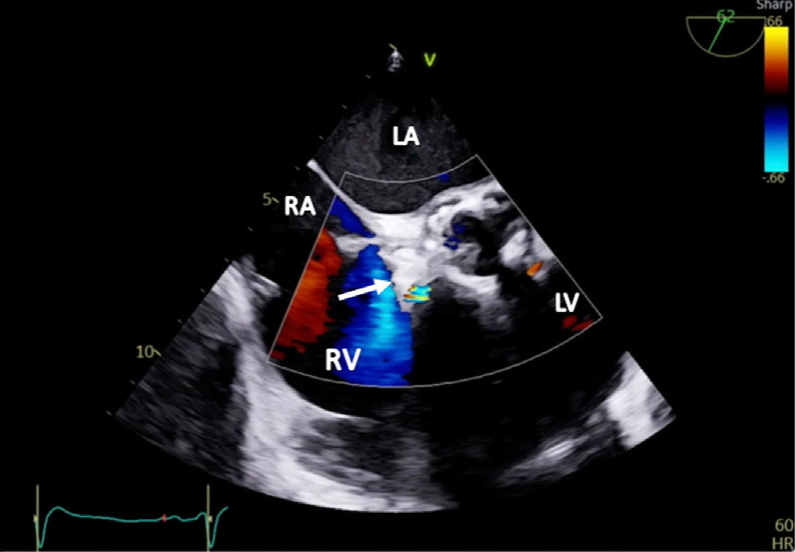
Transesophageal Echocardiographic Short-Axis View at the Aortic Valve Level With Color Flow Doppler Showing Successful Closure of the Right Atrial-Aortic Fistula The arrow shows an occluder device. Abbreviations as in Figures 2 and 3.
